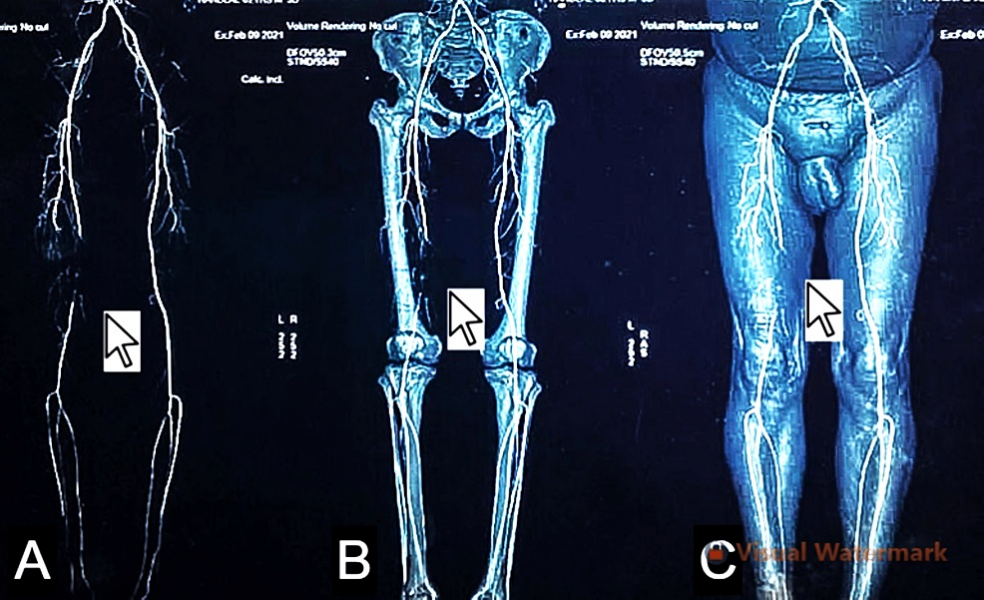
On computed tomography angiography, a complete filling defect of the superficial femoral artery was noted at the level of the mid-thigh with distal recanalization just above the level of the knee, features suggestive of thrombosis, as seen in (A) the reconstruction of arteries without bony and soft tissues, (B) the arterial system in relation to the bones, and (C) the arterial system with bone and soft tissue.
